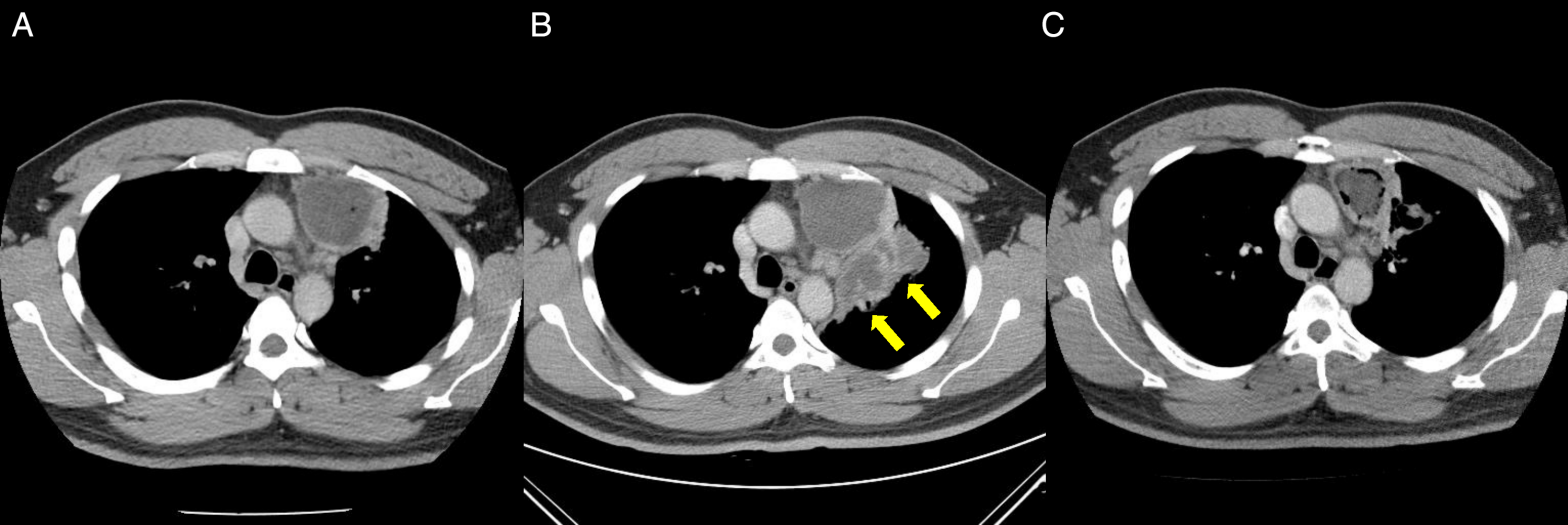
Axial post‐contrast chest computed tomography of a 35‐year‐old man. (A) A cystic heterogeneous lesion over the upper left hemithorax, measuring 7.0 × 6.0 × 5.0 cm. (B) Three weeks later, the lesion enlarged and showed multiloculation, with minimal air bubble sand perforating into the lung and bronchus (arrows). (C) Seven months later, the lesion showed shrinkage in size with irregular consolidation at the upper left lobe of lung.
